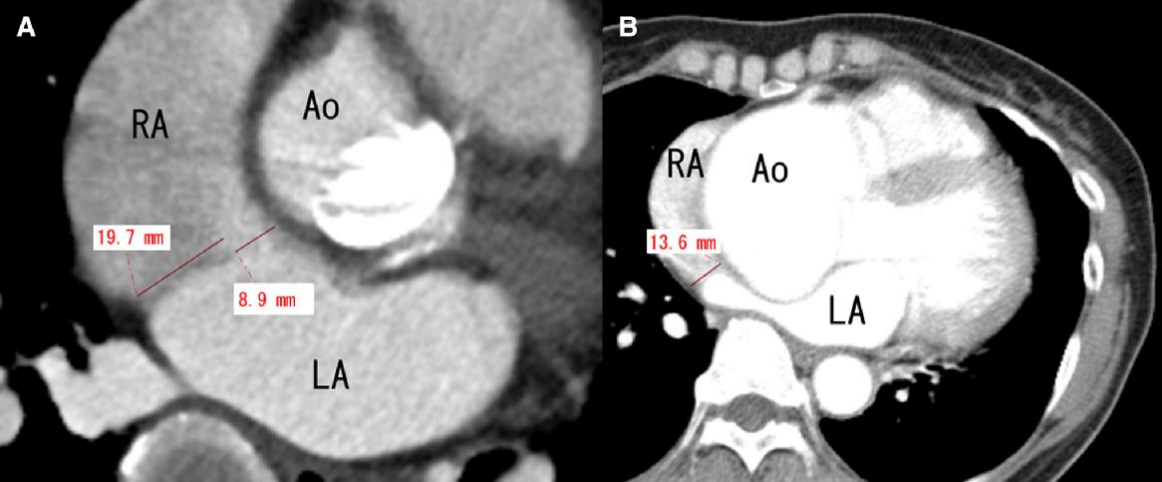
Computed tomography image. (*A*) At the time of secundum atrium septal defect diagnosis. (*B*) Before the aortic root replacement and aortic valve replacement (12 years before the atrium septal defect diagnosis).
